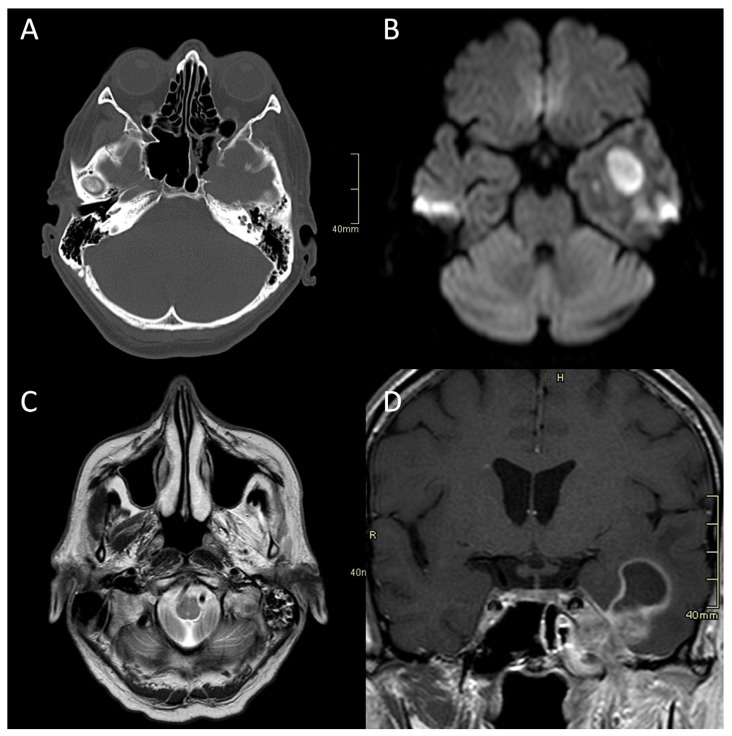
CT and MRI of a patient with fungal atypical skull-base osteomyelitis due to acute invasive fungal sphenoid sinusitis. (A) Axial CT bone algorithm reconstruction showing erosion of the lateral wall of the sphenoid sinus, indicating the spread into the cavernous sinus and middle cranial fossa toward the Meckel’s cave. (B) Axial MRI DWI showing increased signal in the left temporal lobe. (C) Axial MRI T2 showing increased signal in left masticatory muscles due to the denervation corresponding to the damage of the trigeminal nerve. (D) Coronal CE MRI T1 showing the spread of the infection from the sphenoid sinus into the parasellar space, cavernous sinus, and Meckel’s cave, with an intraparenchymal abscess of the temporal lobe. CT: computerised tomography; MRI: magnetic resonance imaging; DWI: diffusion-weighted imaging; CE: contrast-enhanced.
